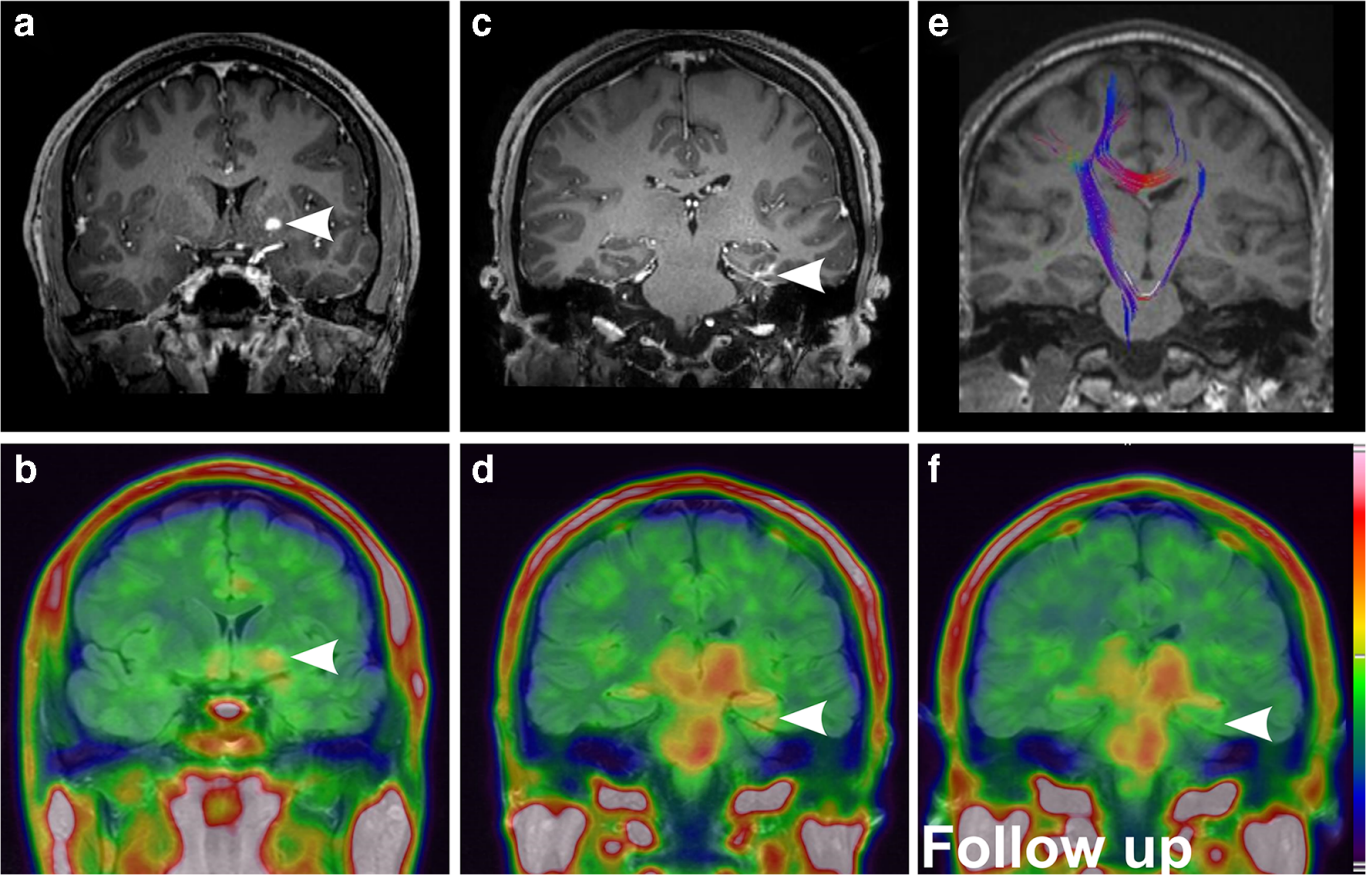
Imaging findings of patient #1 diagnosed with PACNS. Coronal T1wi after Gadovist® injection demonstrates the two major contrast enhancing lesion in the left putamen and left temporal lobe (a, c, arrows, contrast-enhanced MRI 2 weeks before PET scan). Coronal FLAIR/PET overlay demonstrates [^18^F]DPA-714 uptake of the lesions (b, d) with additional regional tracer uptake of the left corticospinal tract. (e) Tractography images overlaid with T1wi demonstrate reduced left sided fiber integrity. Follow-up FLAIR/PET, 4 weeks after induction of anti-inflammatory therapy, (f) demonstrates normalization of uptake in the left temporal lesion and constant uptake of the left corticospinal tract
